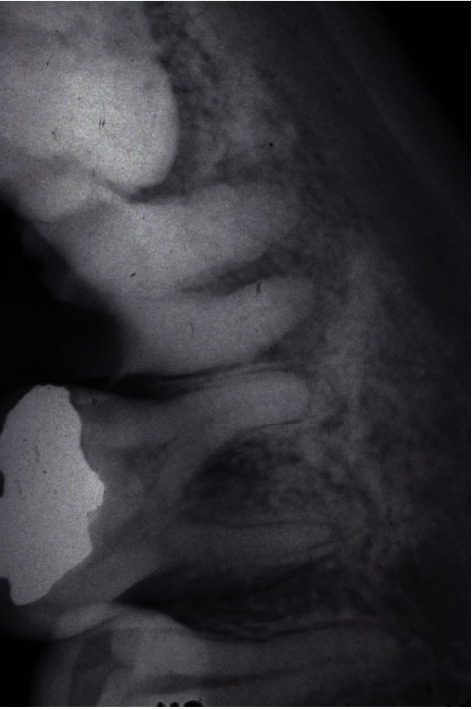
Periapical radiograph at the initial appointment. Large periapical radiolucency was present on the second molar. The gross caries reached to the almost alveolar bone level on the radiograph. This was deemed as a nonrestorable tooth.
